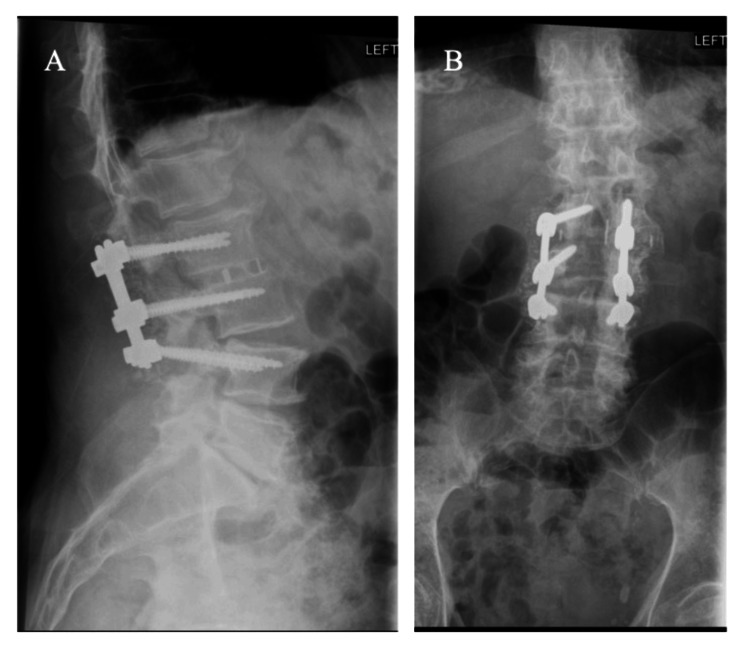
Three months postoperative standing lateral (A) and anteroposterior (C) X-ray shows successful conversion of the cortical screw to the pedicle screw construct without evidence of pseudoarthrosis.
